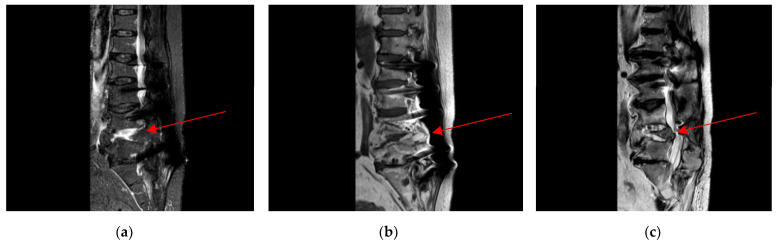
MRI scans in various sequences revealed discal alterations, specifically a hyperintense signal at the intervertebral discs from L3 to L5 on T2-weighted sequences, without evidence of involvement of the adjacent vertebral bodies or surrounding soft tissues (red arrows). Progressive fusion was observed at the residual L4–L5 disc space, associated with right-sided neuroforaminal stenosis. (a) Lumbosacral magnetic resonance imaging using STIR sequences. (b) Lumbosacral magnetic resonance imaging using T1-weighted sequences. (c) Lumbosacral magnetic resonance imaging using T2-weighted sequences.
